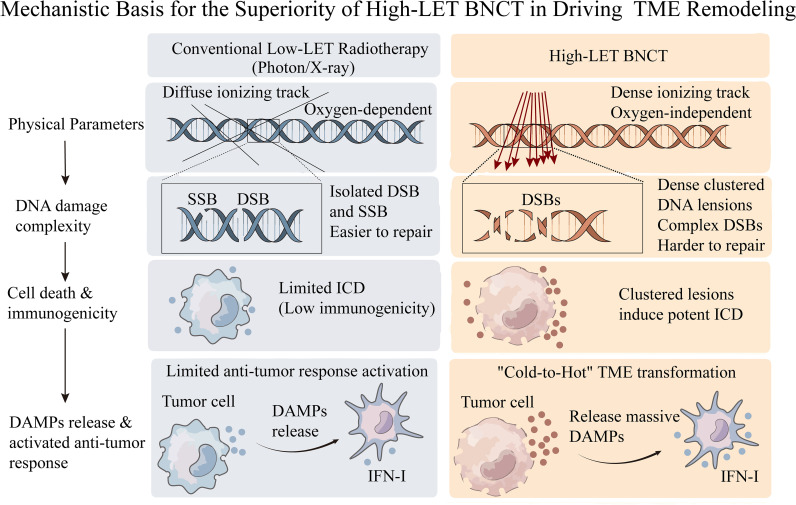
Mechanistic basis for the superiority of high-LET BNCT in driving TME remodeling. Different physical properties between low-LET RT and high-LET radiation induce difference in DNA damage complexity, cell death immunogenicity, and anti-tumor immune activation. Low-LET radiotherapy (photon/X-ray) induces diffuse, oxygen-dependent ionization and forms repairable isolated DNA lesions, leading to limited ICD and restricted anti-tumor responses. In contrast, high-LET BNCT generates dense, oxygen-independent ionizing tracks and induces irreparable clustered DNA lesions, triggering potent ICD, robust DAMP release, and IFN-I production, thereby driving the transformation of immunosuppressive “cold” TME into immune-activated “hot” TME, ultimately potentiating systemic anti-tumor immunity.
